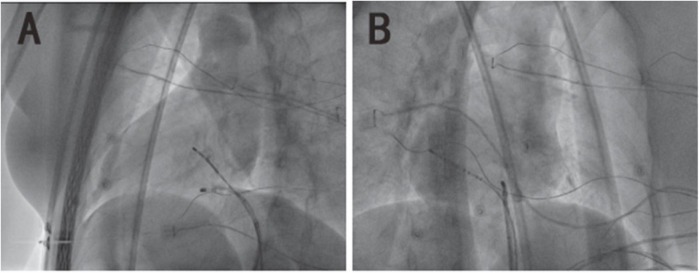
The X-ray image of mirror dextrocardia in the left anterior oblique view of 45° and right-anterior oblique view of 35°. (A) The image of dextrocardia in the left anterior oblique is presented as a common heart in the regular right anterior oblique. (B) The image of dextrocardia in the right anterior oblique is presented as a common heart in the regular left anterior oblique.
